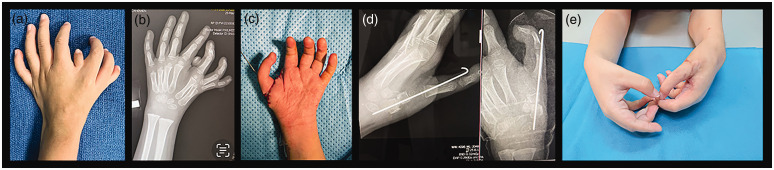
Case 20. (a) Polydactylous hand; (b) radiograph shows seven triphalangeal rays and one biphalangeal ray; (c) after transfer of ‘best’ digit to most preaxial metacarpal base, age 3 years; (d) postoperative radiographs and (e) both hands at age 4.2 years.
